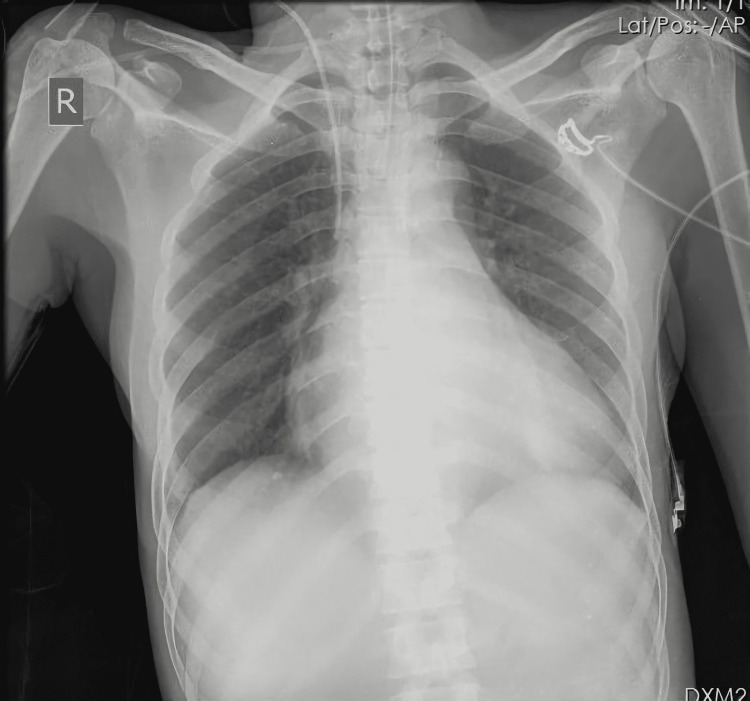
Postoperative chest X-ray
